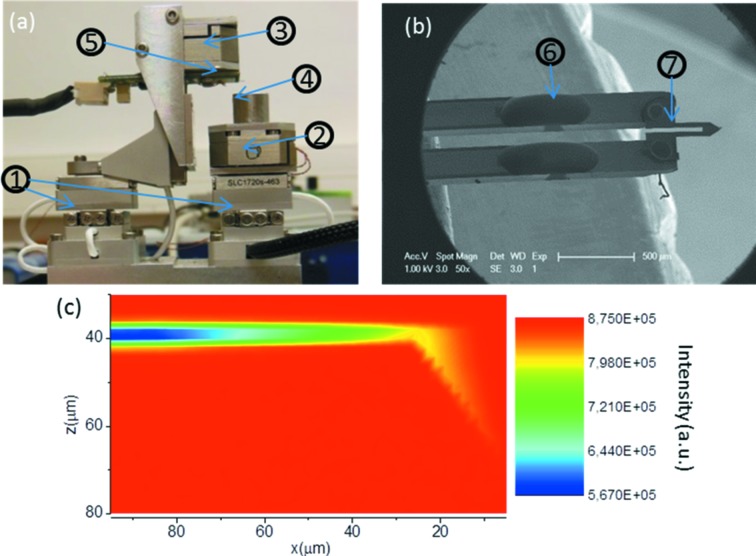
(*a*) Photograph of SFINX which contains (1) two stacks of long-range piezo stages, (2) *xy*-piezo scanner, (3) *z*-scanner, (4) sample stage and (5) AFM cantilever. (*b*) SEM image of the Akiyama probe consisting of (6) a quartz tuning fork and (7) a Si tip. (*c*) Scanning X-ray transmission map of the AFM tip.
